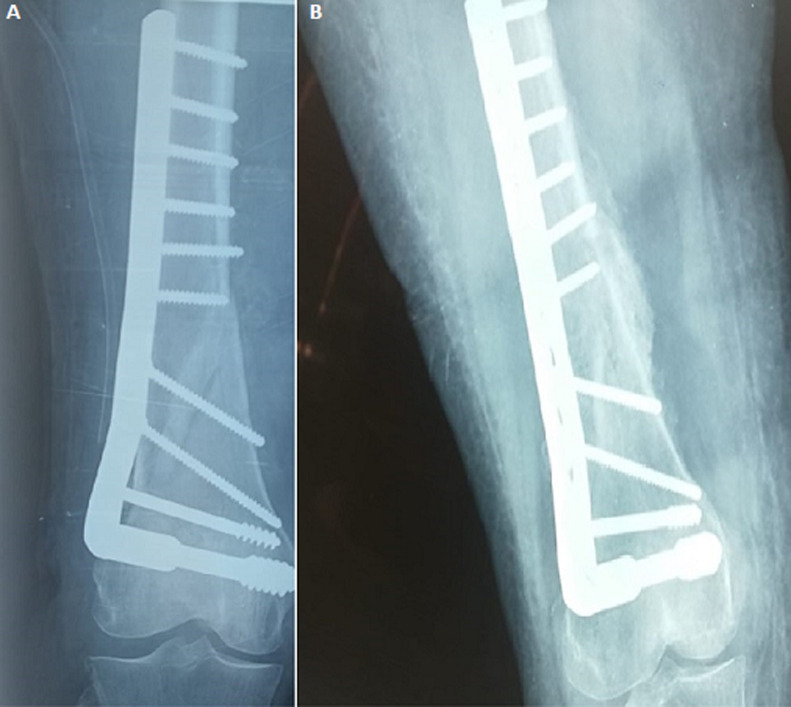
anteroposterior view X-rays showing good callus formation at end of four months (A) and six months (B) in an extra-articular distal femur fracture
